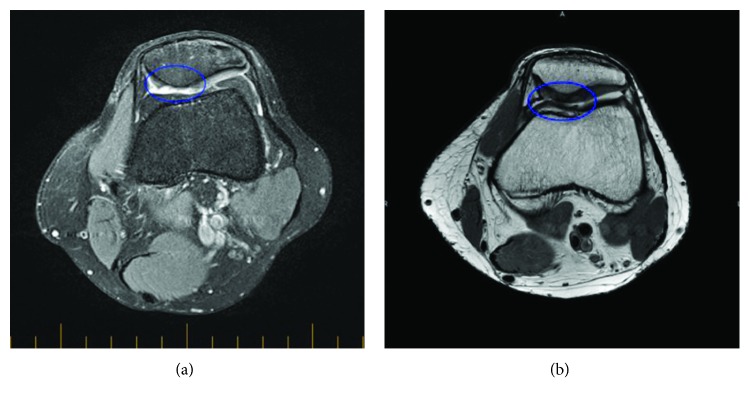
(a) Pretreatment proton density fat-suppressed axial MRI of the knee showing the isolated chondral defect involving the medial facet of the patella. (b) Posttreatment proton density axial MRI indicating articular cartilage regeneration at the site of the chondral defect with smooth integration with the surrounding joint surface [75].
